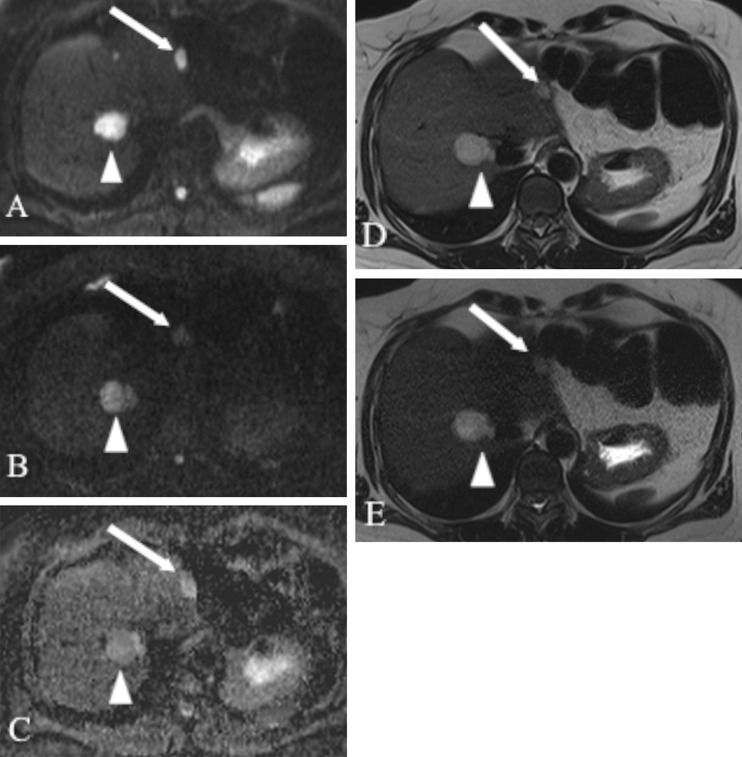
MR images obtained in a 53-year-old woman with hepatic haemangiomas. a
